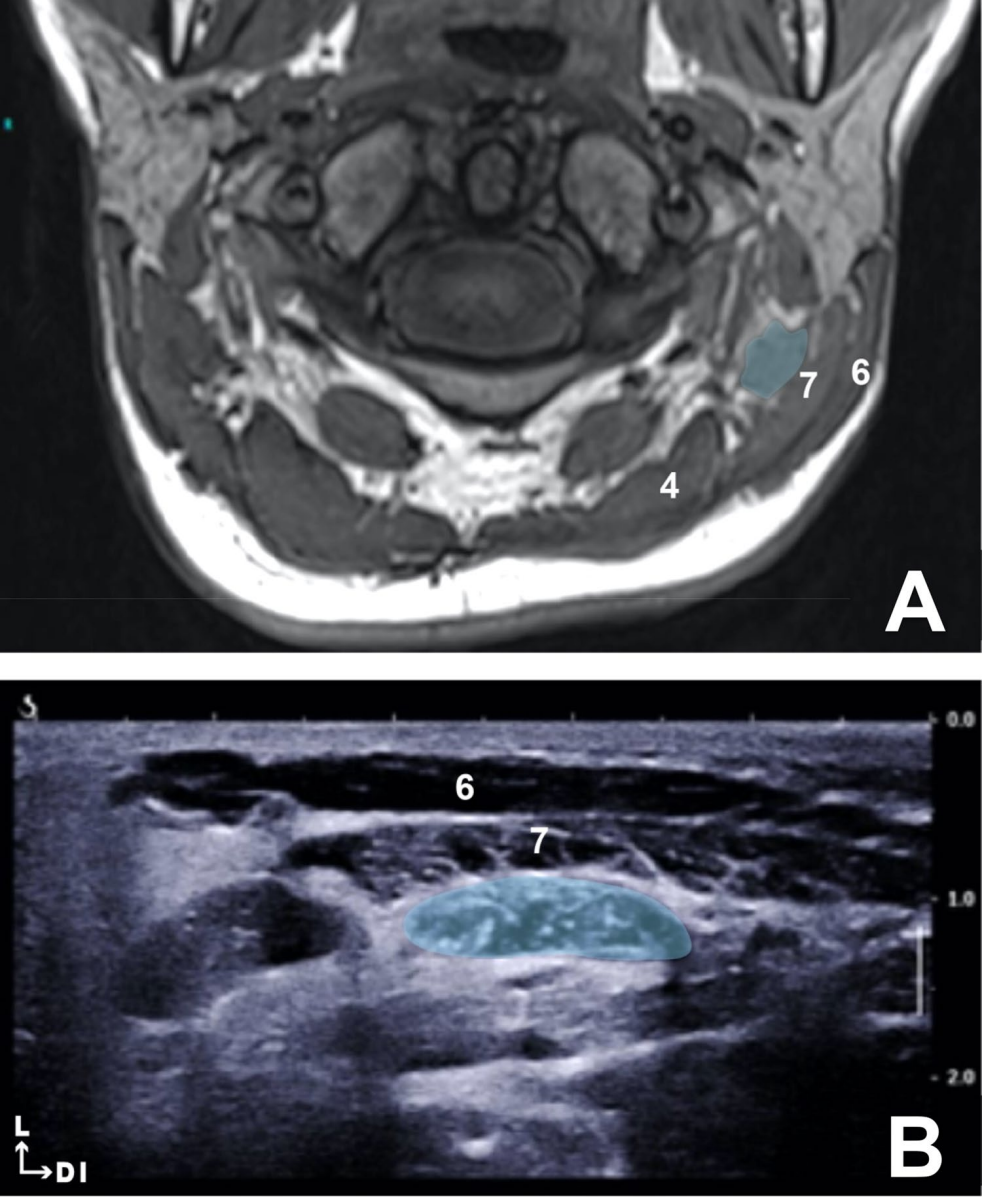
a T1-weighted MRI, axial slice at level of the dens of axis. Longissimus capitis (blue). Sternocleidomastoid muscle (6), splenius capitis (7), and semi-spinalis capitis (4). b Ultrasound image at the level of the dens of axis. Longissimus capitis (blue). Sternocleidomastoid muscle (6) and splenius capitis (7)
